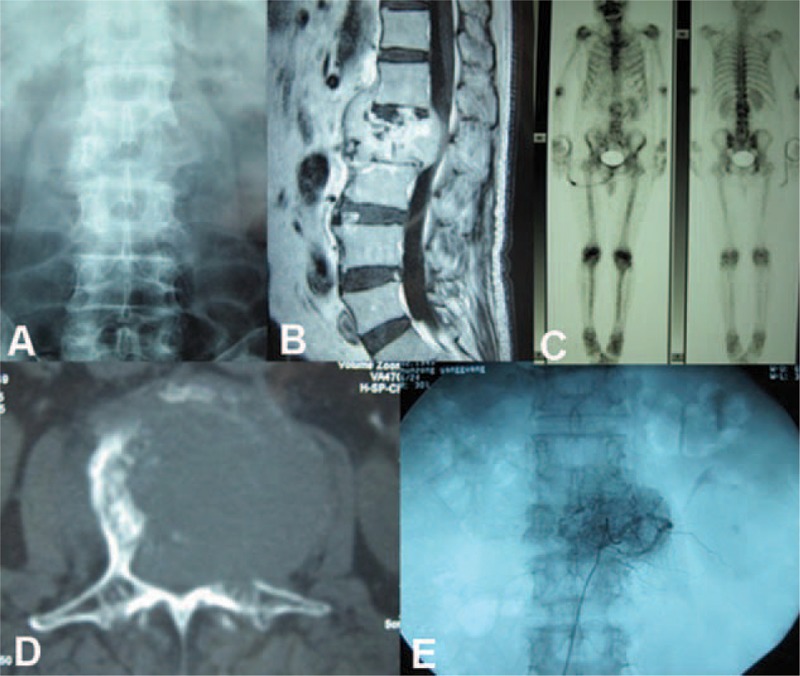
(A) Preoperative radiography. (B) MRI. (C) Bone scintigraphy. (D) CT. (E) Preoperative vertebral tumor embolization.
